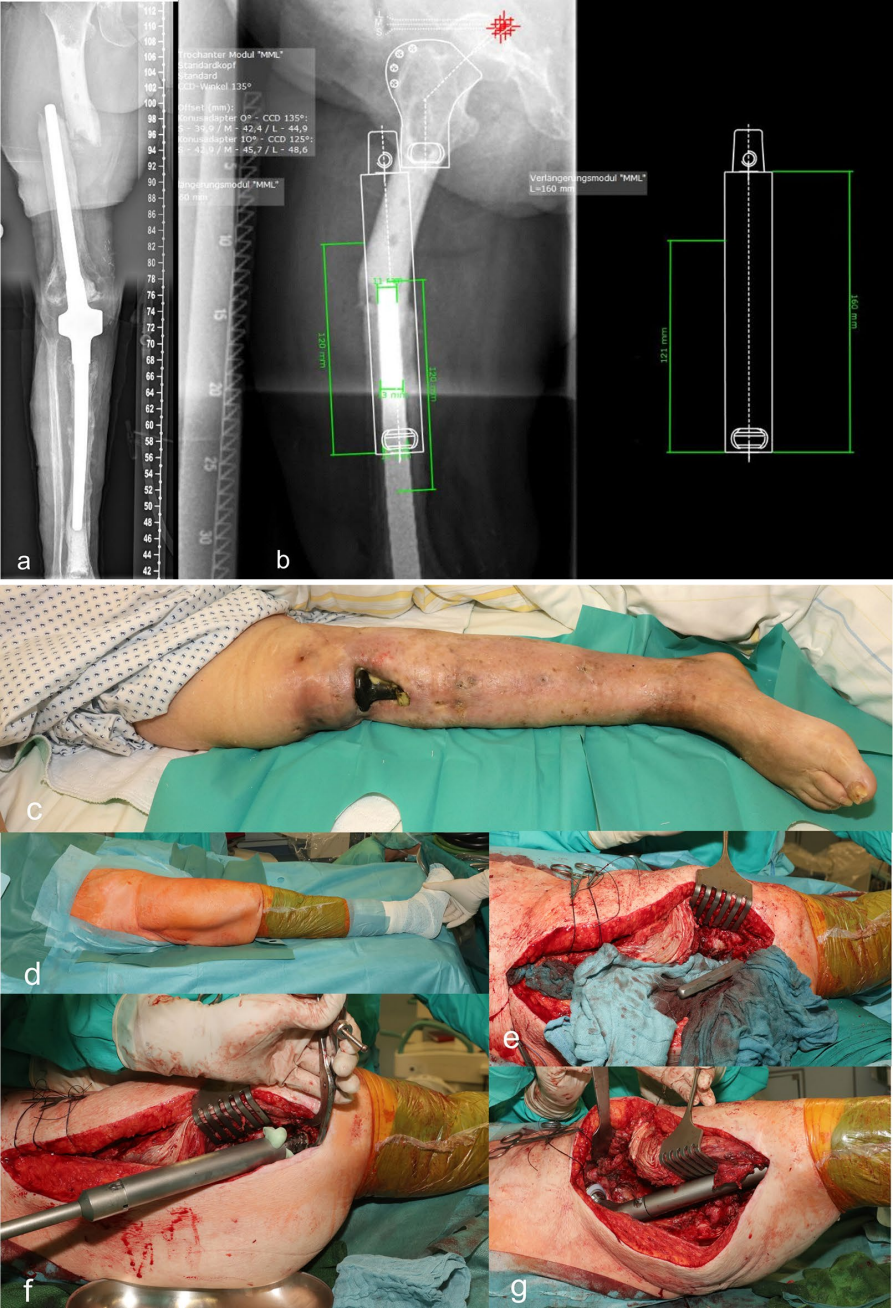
X-ray of a female patient, who presented with a periprosthetic fracture of the proximal femoral shaft (a). The patient was suffering from a chronic infection of the intramedullary knee arthrodesis after previous surgeries with a soft tissue defect and longstanding open wound at the ipsilateral knee (c). Special preoperative computerized planning for the off-label use of the modular component from a different company was necessary (b). Intraoperative pictures of the preparation of the arthrodesis stem (e), off-label fitting of the modular component on the fixed stem using bone cement (f) and the final reduction in situ (g). The side of the infected arthrodesis of the knee was covered at all time (d). Postoperative antero-posterior x-ray imaging of the fitted implant after resection and replacement of the proximal femur (e). Postoperative clinical findings with a proximal wound after implantation of a proximal femur replacement with no signs of local infection and the existing soft tissue defect of the knee (f). On a clinical and radiological check-up more than six months after surgery, the patient presented mobile without the use of crutches for a limited distance in her home with total weight bearing on the right leg. As expected, we saw clinical signs of infection at the proximal implant site as well as a raise in C-reactive protein (g).
